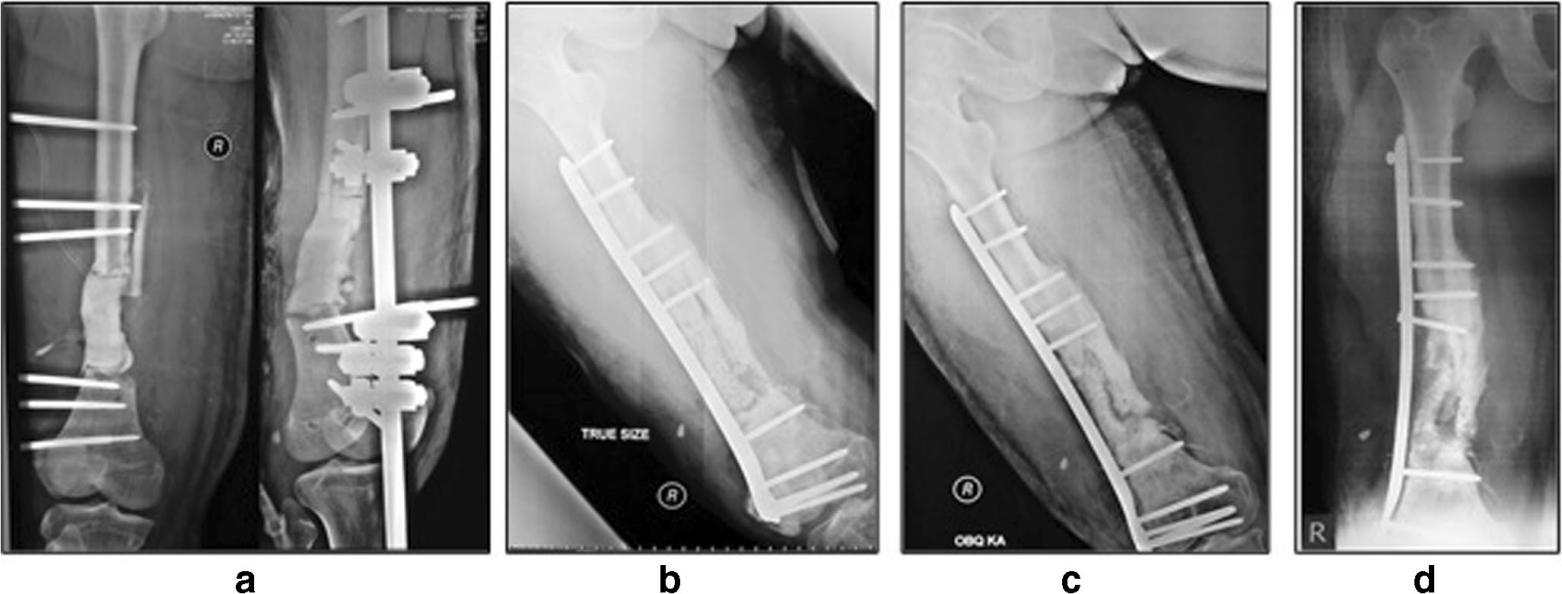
A 34-year-old male (case 2) diagnosed with infected open fracture of the right distal femur with 7-cm bone defect after Masquelet procedure. a Pre-operative radiography. b Post-operative radiography, c Twelve-month radiography. d Twenty-one-month radiography
